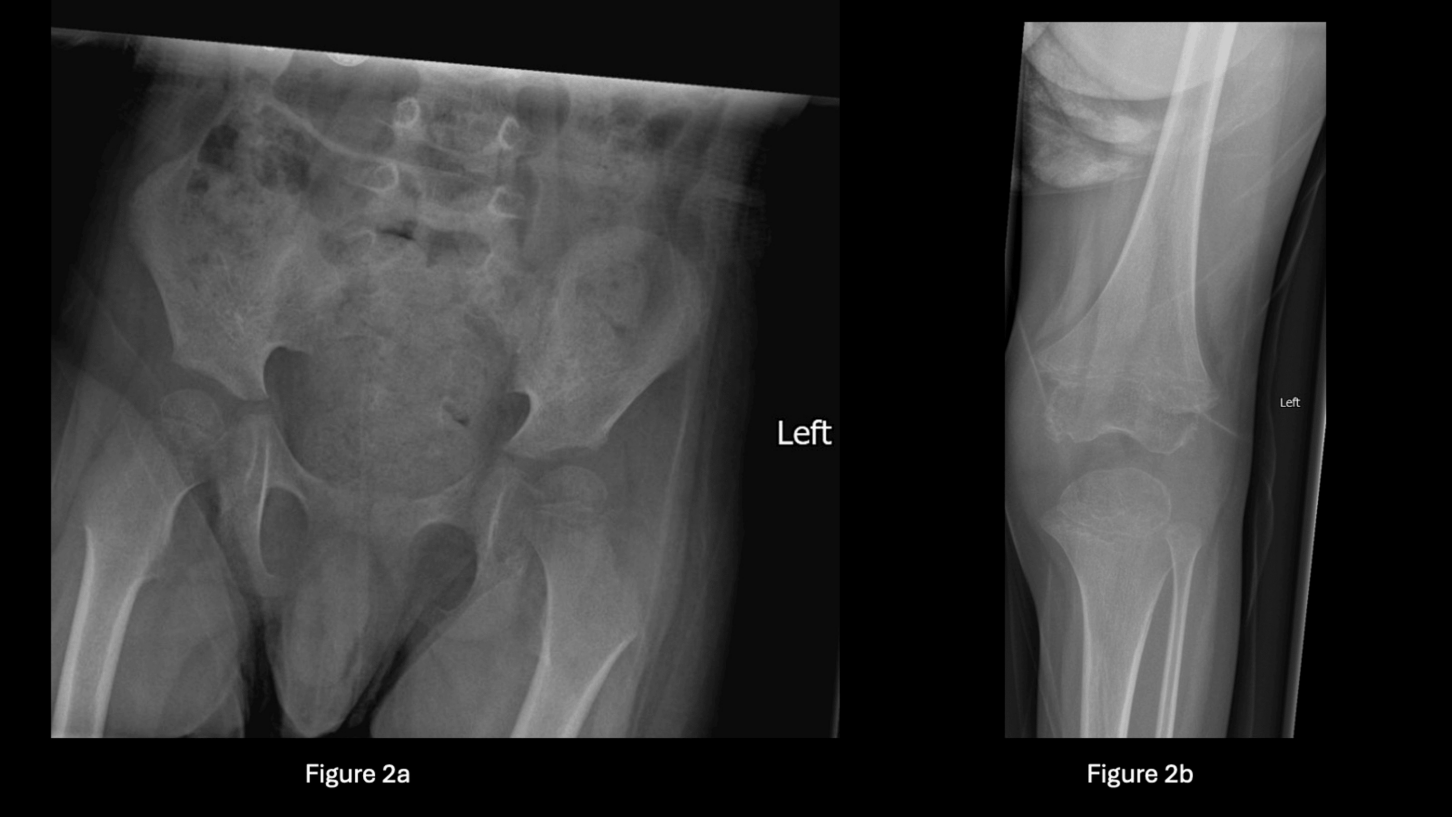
X-rays of pelvis and left knee on follow up in 3 weeks' time Figure 2a is the pelvis and Figure 2b is the left knee. No obvious abnormality was noticed on the pelvis. However, irregularities were seen in the left knee epiphysis.
